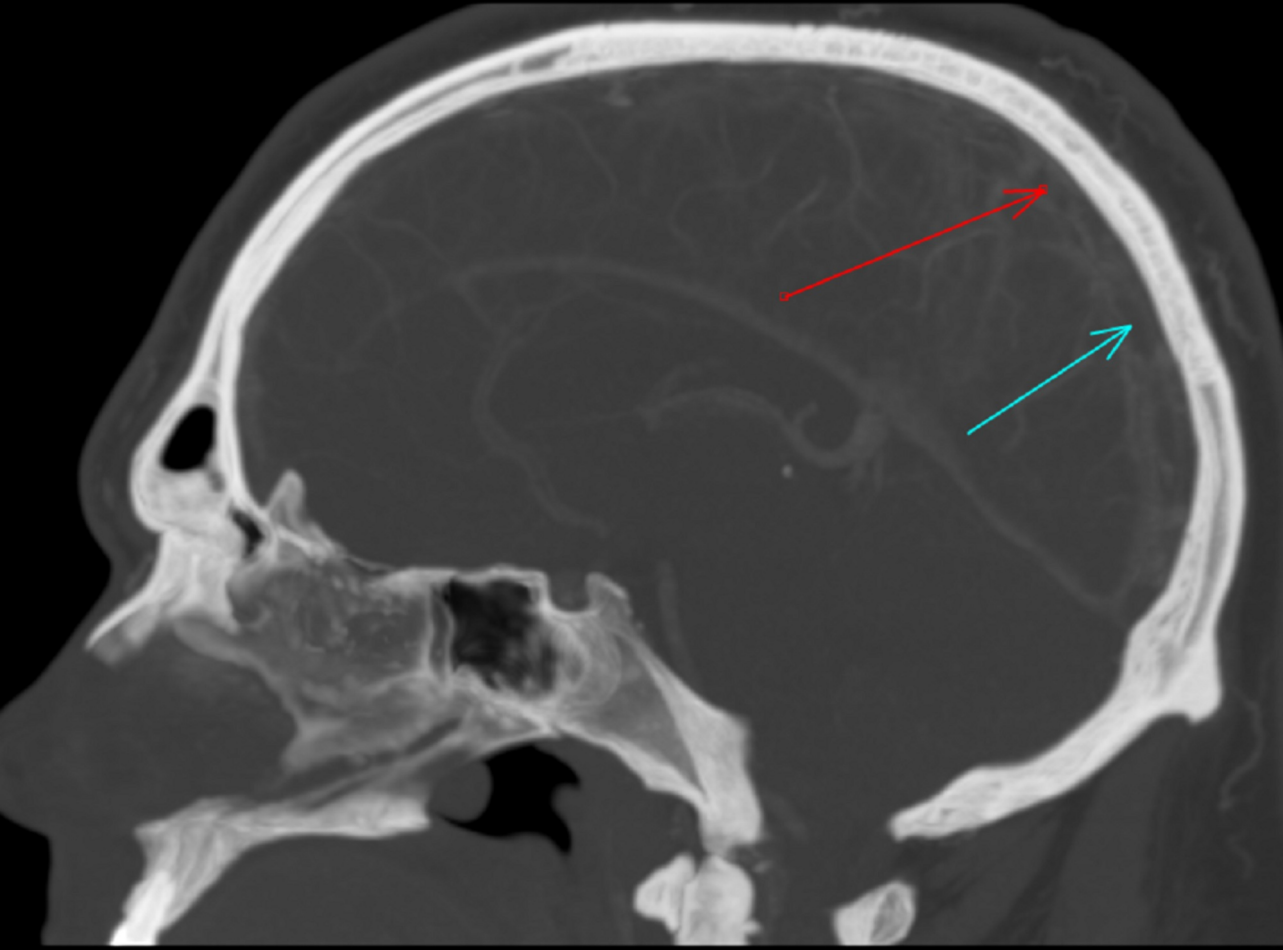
Pre therapy computed tomography with contrast, arrows showing superior venous thrombosis
